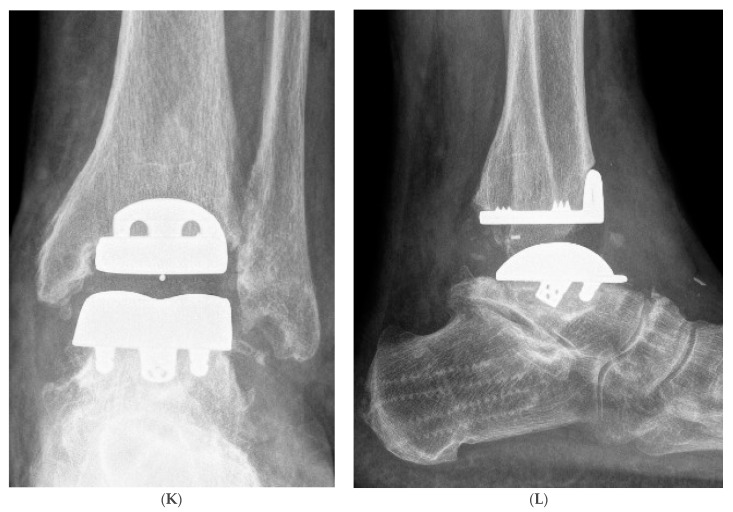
Hybrid Total Ankle Arthroplasty (H-TAA). This figure represents Case Nr. 7 of the cohort (see Table 1, Table 2 and Table 3). (A–D): Preoperative X-rays and CT-scans depicting the cyst under the talar component of the Hintegra TAA. (E,F): Intraoperative pictures showing the inlay breakage with poor bone osteointegration in the backside of the talar component of the Hintegra TAA. (G): Talar cyst with stable tibial Hintegra component. (H): Performance of a flatcut of the talus and bone grafting of the talar cyst with iliac crest autologous spongiosa. (I): Flatcut Talar Component of the VANTAGE TAA, which is implanted on the reconstructed talus. (J): H-TAA: Tibial component Hintegra TAA with new Flatcut Talus and Inlay of VANTAGE TAA. (K,L): Postoperative X-rays with H-TAA, good talar bone reconstruction, and good integration of the Flatcut Talus of VANTAGE TAA.
